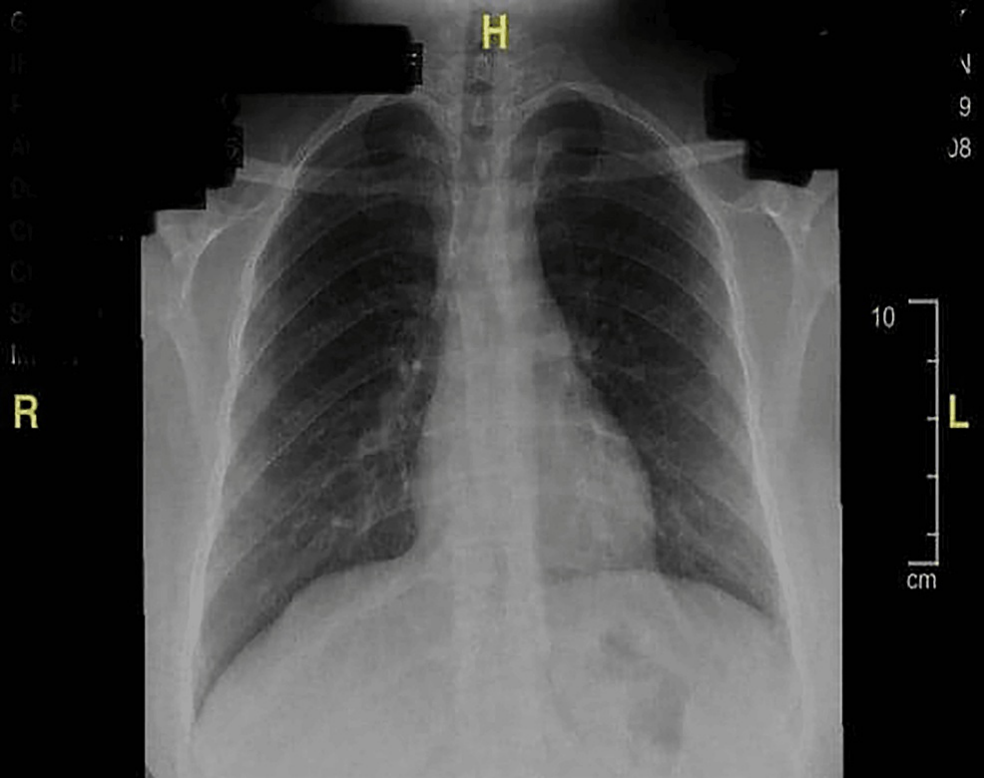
Chest X-ray of the patient conducted in October 18, 2019.
